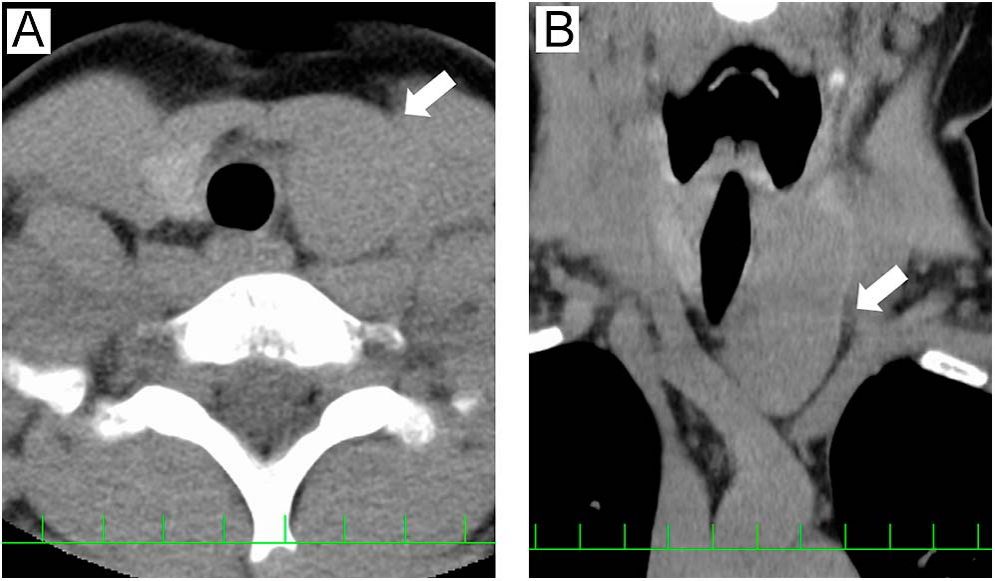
(A) CT scan showed homogeneous and hypodense mass on the left side of the trachea. (B) The lower pole of the tumor extended into the mediastinum behind the sternum.
